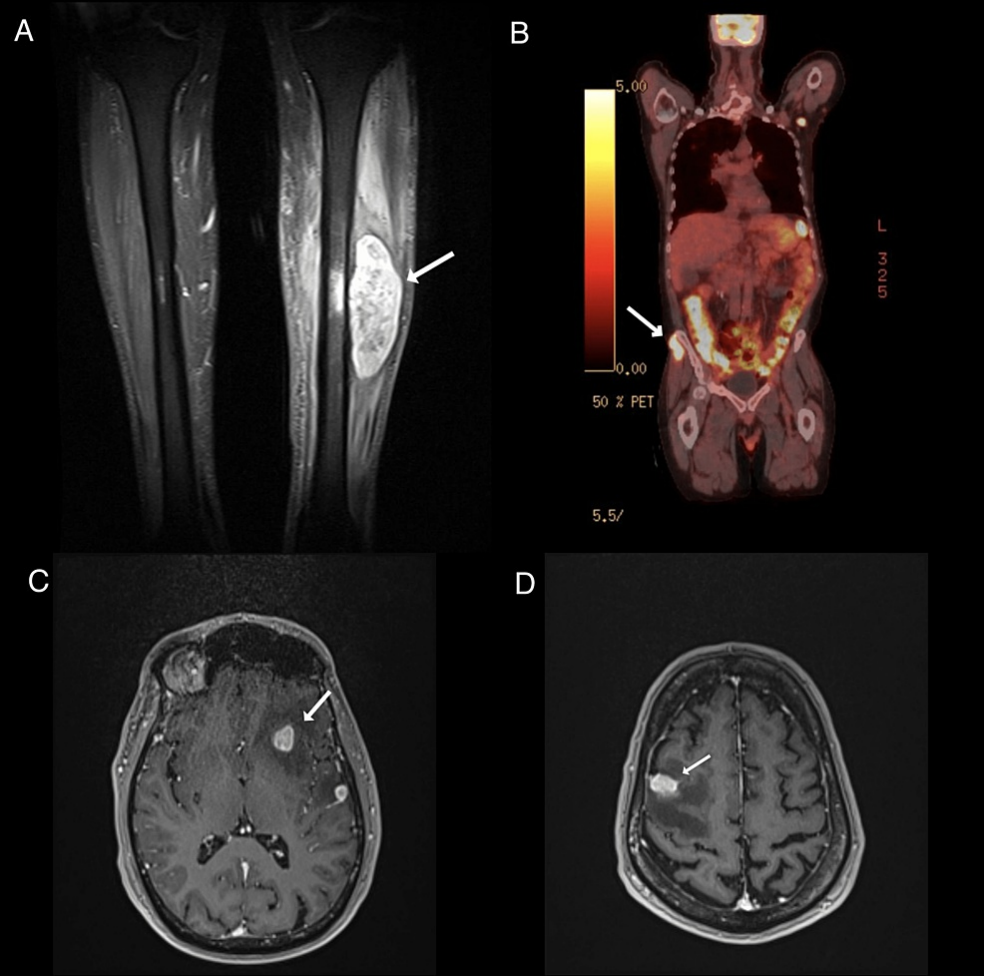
MRI of the left leg showing Intramuscular mass in the tibialis anterior muscle (A). FDG-PET scan showing 2.6 cm hypermetabolic soft tissue mass in the subcutaneous fat and gluteus medius along the right iliac crest (B). MRI of the brain shows enhancing lesions in the brain's left (C) and right (D) hemispheres. MRI - magnetic resonance imaging; FDG-PET - 18-Fluorodeoxyglucose positron emission tomography
